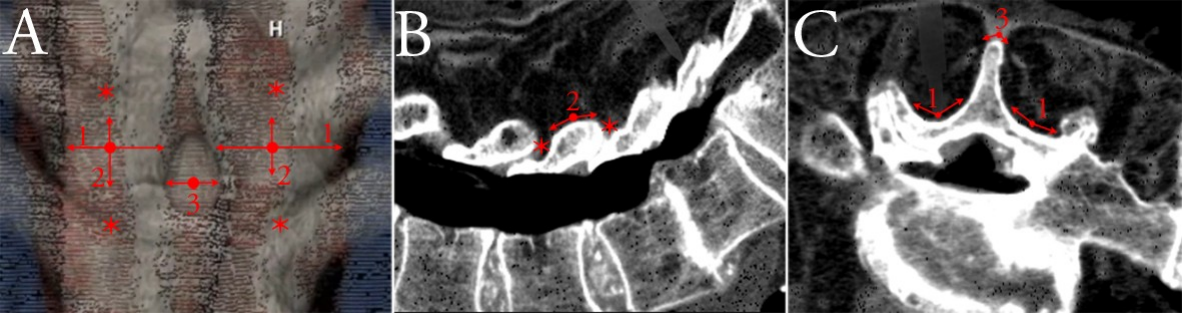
(A) Representative point cloud of an open bilateral posterior lumbar exposure. Manual accuracy verification should be performed with a tracked sharp-tip tool statically at the superior and inferior facet joints (*), and dynamically by sliding axially (1) and sagittally (2) along the hemilaminae as well as along the spinous process tip (3). Verification steps shown on orthogonal sagittal (B) and axial (C) CT reconstructions as seen on typical navigation displays, with static verification points (*) and dynamic sliding maneuvers (1, 2, 3).
